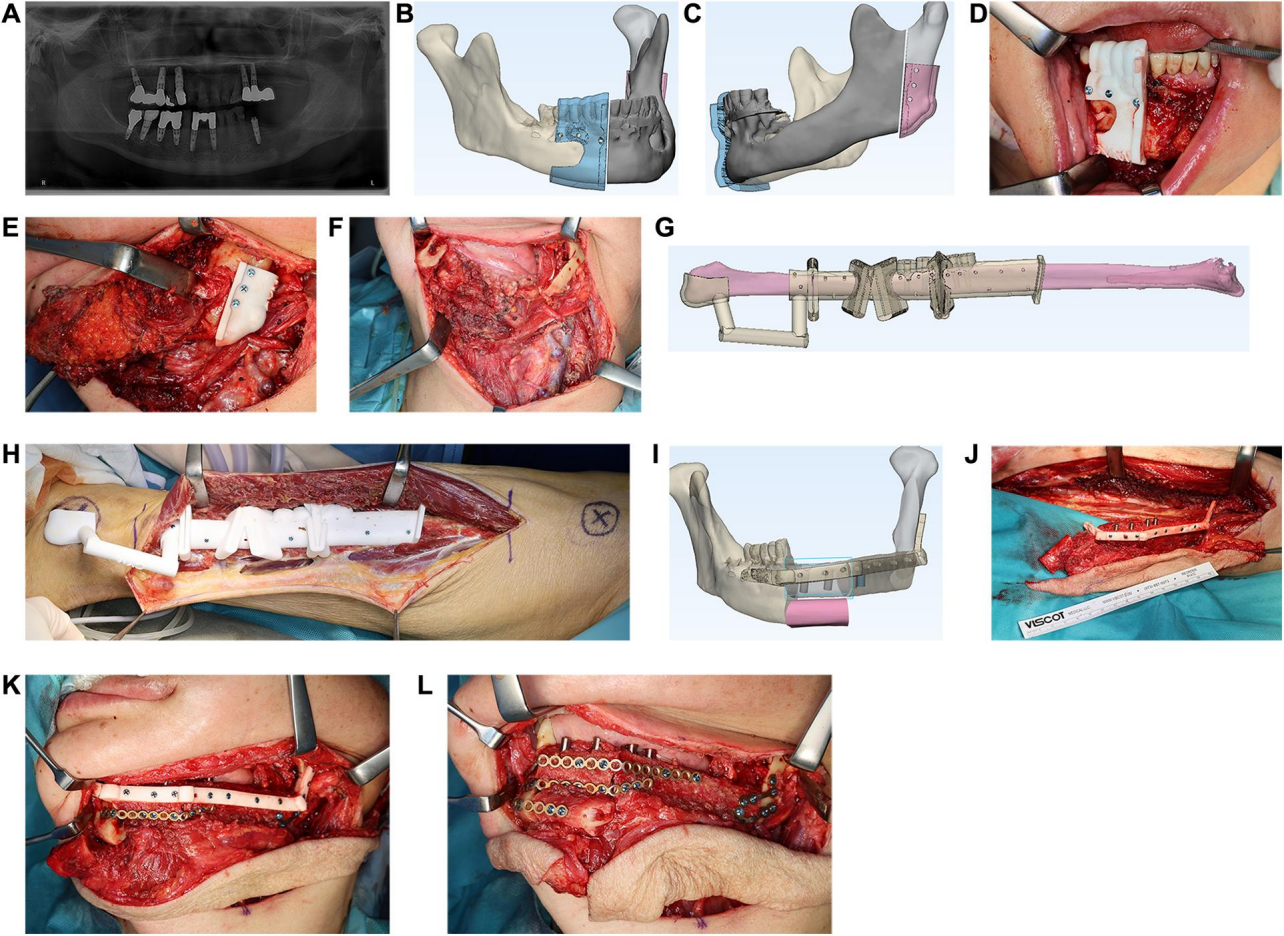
Mandibular reconstruction with fibula free flap after partial mandibulectomy for intraosseous squamous cell carcinoma. (A) Orthopantomogram demonstrating an osteolytic lesion at the left mandible involving the mandibular canal. (B,C) VSP of the mandibular resection guide. (D,E) Mandibular resection guide fitted intraoperatively with screws. (F) Mandibular defect following guided resection. (G) VSP of fibula segmentation guides with an in-built implant placement guide. (H) Fibula segmentation guide fitted intraoperatively with screws. (I) VSP of the double-barrel mandibular reconstruction and TPSP. (J) Assembly of the neomandible with TPSP at the donor site prior to dividing the pedicle. (K) Fibula free flap transferred and temporarily stabilized with TPSP to enable fixation of conventional miniplates. (L) Final fixation of fibula segments following removal of TPSP.
